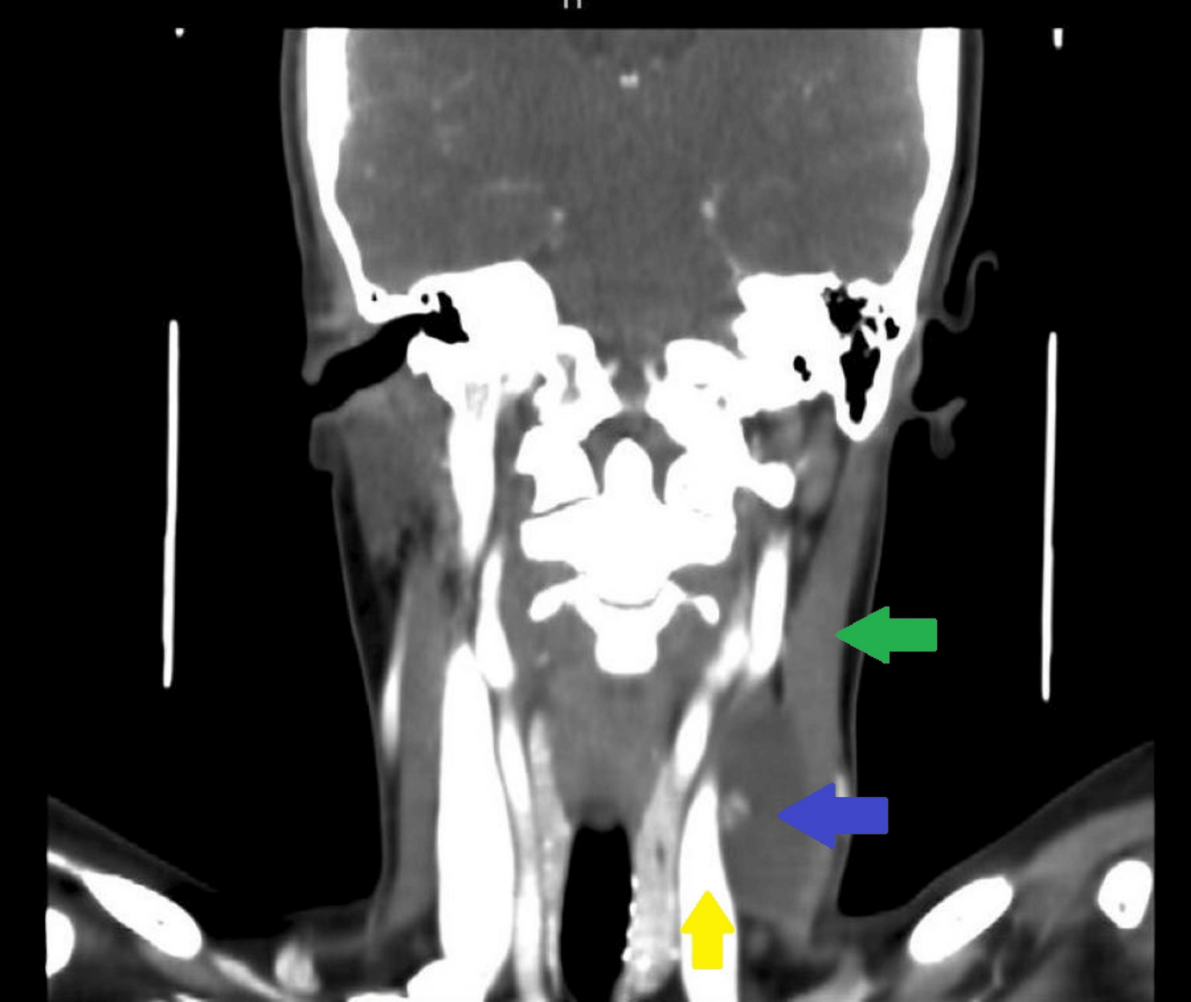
Computed tomography of the neck The sagittal view showed a well-defined left deep cervical cystic lesion with solid components measuring about 1.7x3x4.7 cm (blue arrow). The lesion exhibits a deep insinuation between the left sternocleidomastoid (green arrow) and jugular vein (yellow arrow).
